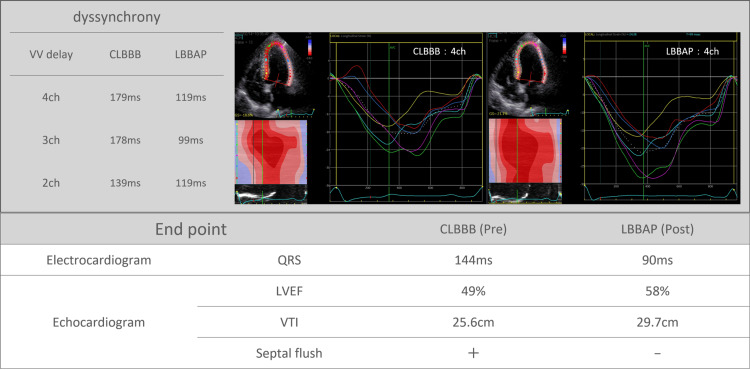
Speckle tracking analysis of mechanical dyssynchrony by echocardiography. LBBAP eliminated septal flash, improved dyssynchrony throughout ventricular activation phases. LVEF: left ventricular ejection fraction; VTI: velocity-time integral; CLBBB: conventional left bundle branch block; LBBAP: left bundle branch area pacing
